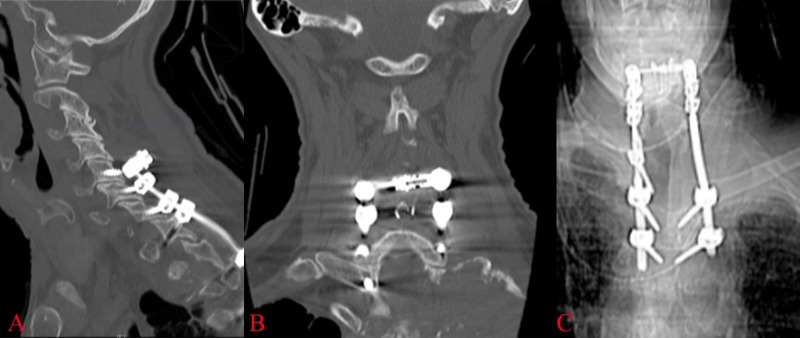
Post-fall imaging A post-fall CT scan in sagittal (A) and coronal (B) sections demonstrates hardware integrity with no evidence of pull out at the top of the construct. (C) An anterior-posterior x-ray demonstrates an intact construct in this view.
